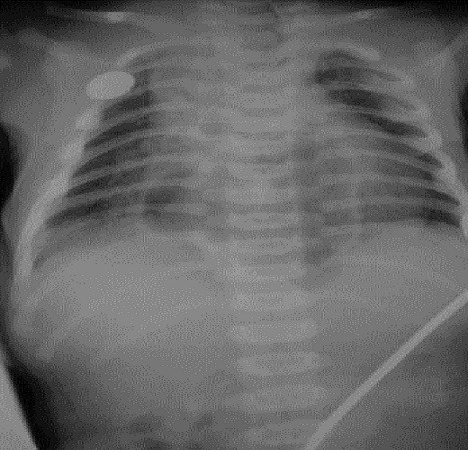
Neonatal chest X-ray showing catheter in esophagus at T6 vertebral level and intrathoracic gastric bubble in the mediastinum
